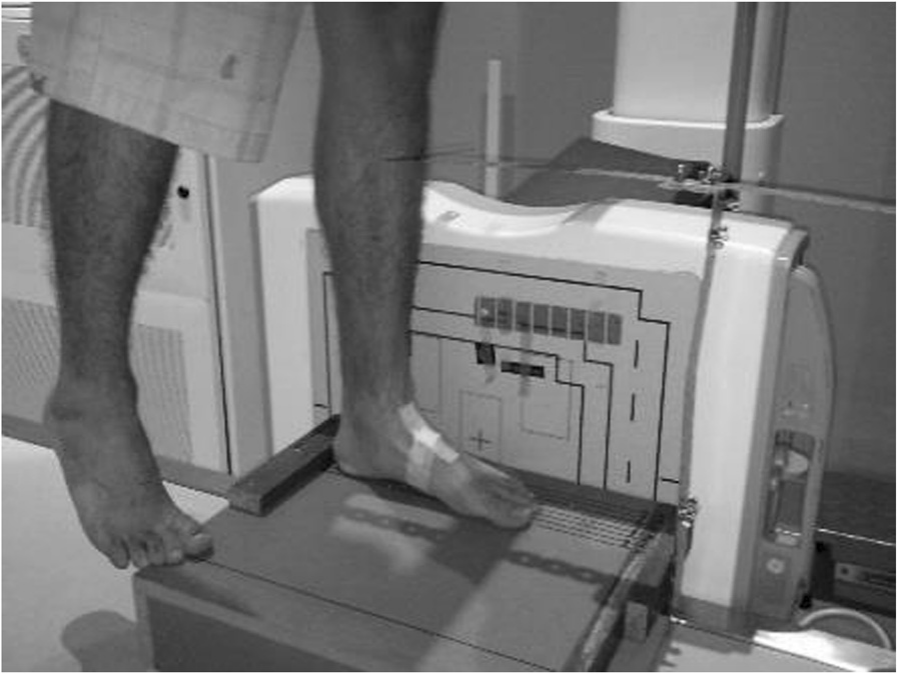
Platform constructed in order to standardize position of foot and leg relative to the X-ray camera and plate.
